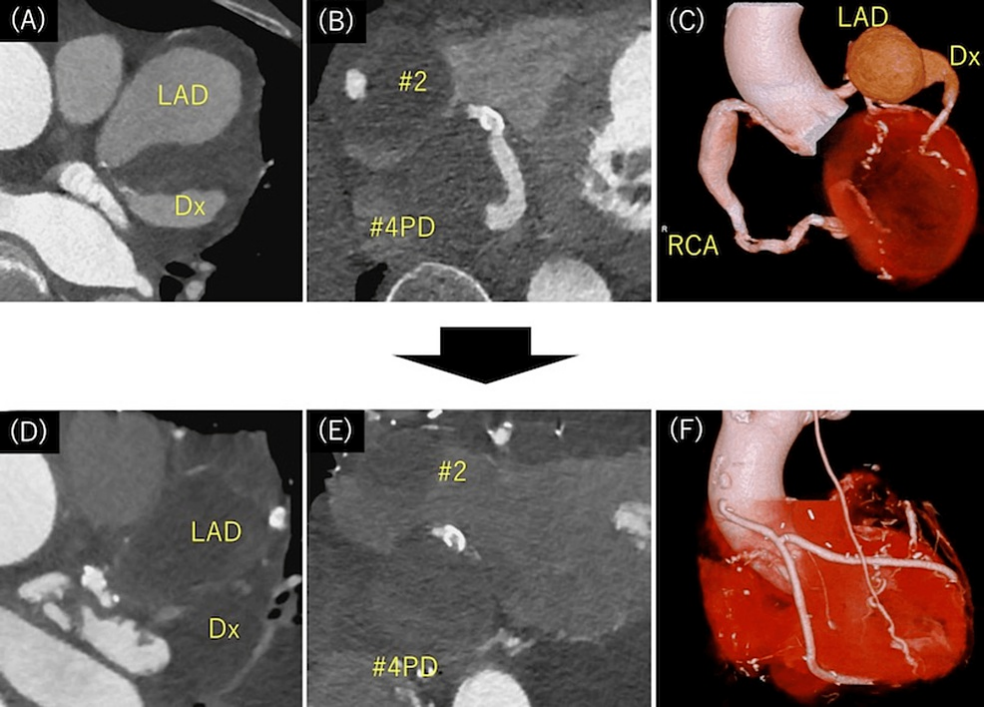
Preoperative (A-C) and postoperative (D-F) cardiac computed tomography (A, B) Preoperative horizontal view. (C) 3D image; LAD aneurysm was close to the LMT. (D, E) Postoperative horizontal view; there was no blood flow in all CAAs. (F) All grafts were patent. LMT: left main trunk; LAD: left anterior descending; Dx: diagonal branch; PD: posterior descending; RCA: right coronary artery; CAA: coronary artery aneurysm
